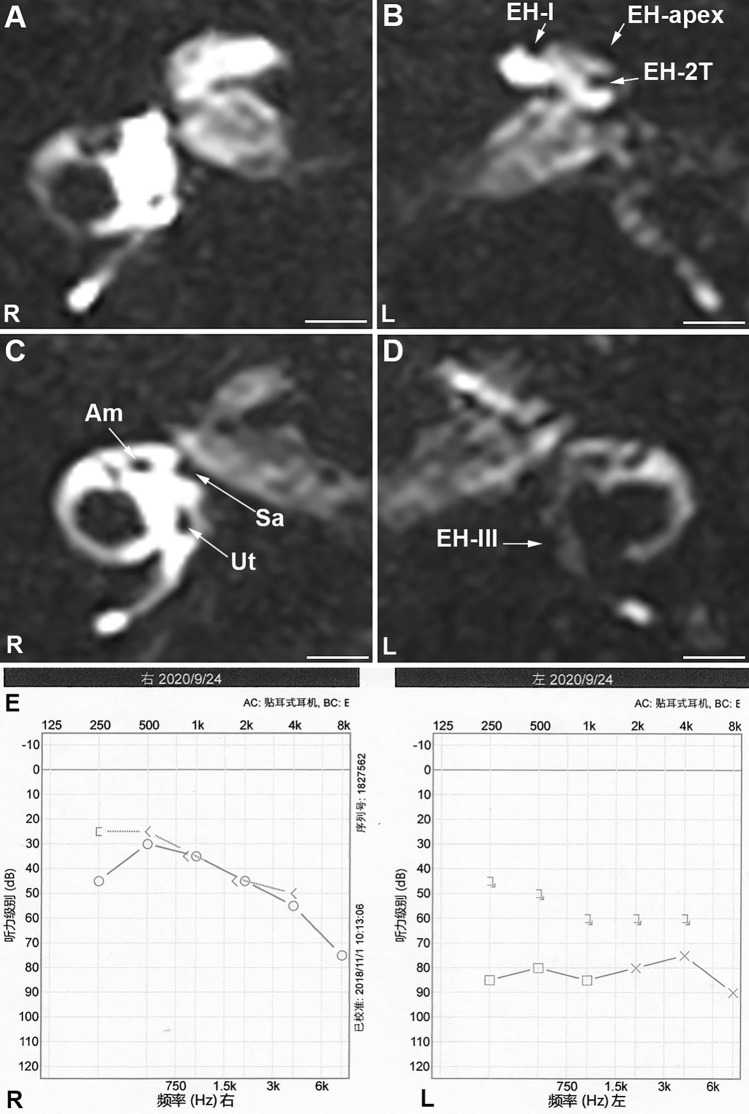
MRI of a patient with bilateral Meniere’s disease imaged using hT_2_W–FLAIR–MZFI 24 h after targeted delivery of 20-fold-diluted Gd–DTPA. A 57-year-old female had a history of over 12 years of episodic vertigo lasting for 3 h accompanied by fluctuating left ear hearing loss and bilateral tinnitus and aural fullness. The diagnosis was definite Meniere’s disease on the left side and probable Meniere’s disease on the right side. The endolymphatic hydrops (EH) was grade I in the left (L) cochlea B and grade III in the L vestibule D. EH was also detected in the L apex (EH-apex) that formed an invaginated arc and second turn (EH-2 T) B. No EH was detected in the right (R) ear A, C. There was profound hearing loss in the left ear and age-related hearing loss in the right ear E. *Am* ampule, *Sa* saccule, *Ut* utricle. Scale bars = 3.0 mm
